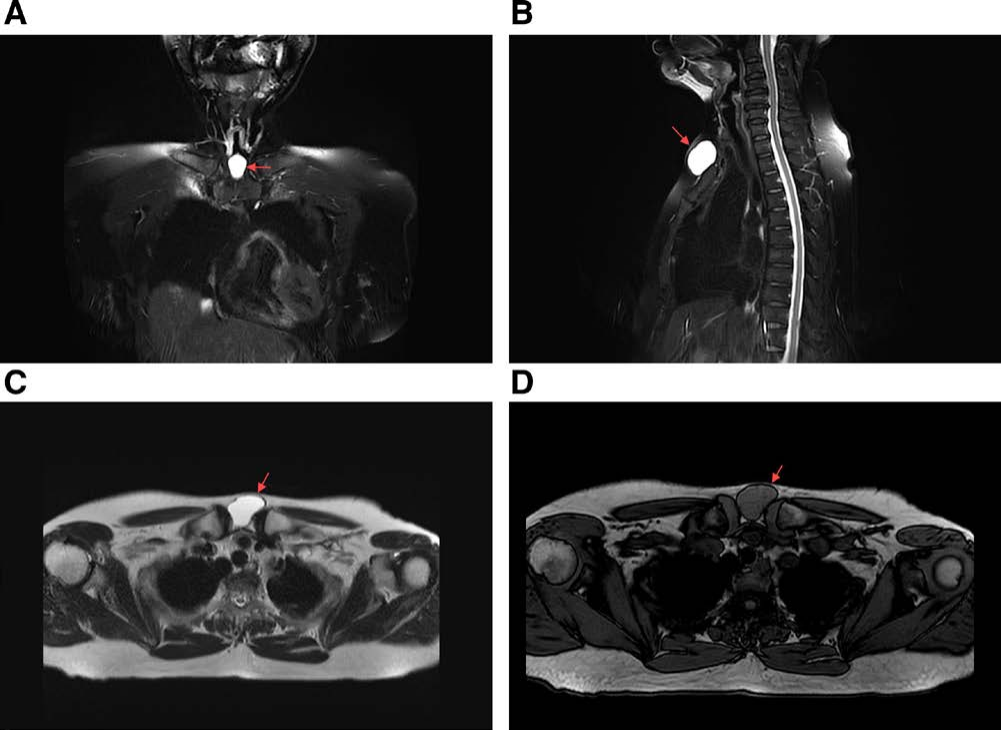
The cyst’s imaging anatomy was clearly identified through neck anteroposterior (A) and lateral (B) MRI examinations. Multiplanar magnetic resonance imaging findings demonstrated a cystic lesion exhibiting hypointense signals on T2-weighted imaging (C) and marked hyperintensity on T1-weighted sequences (D). The cystic mass was consistently demarcated by red arrows across all imaging series.
